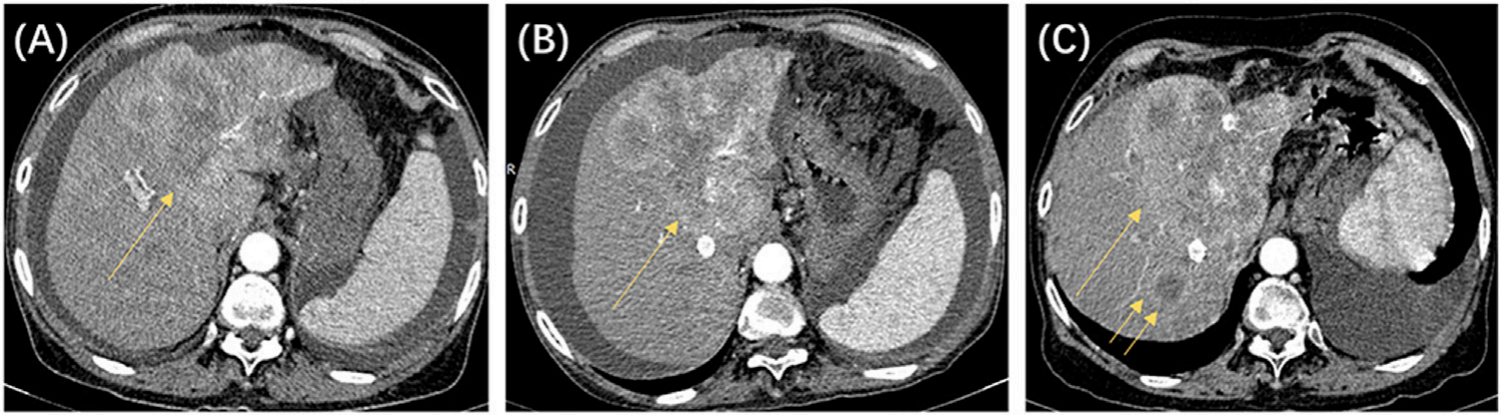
The patient was a 64-year-old woman, with a clinical diagnosis of hepatocellular carcinoma, Barcelona Clinic Liver Cancer stage B, and pre-treatment hepatic function of the Child–Pugh grade (B). The above shows the contrast-enhanced CT images before and after locoregional interventional therapy. (A) Image taken before the initial drug-eluting beads transcatheter arterial chemoembolization (DEB-TACE) procedure, showing that the left liver lobe is full of blood-supplying, space-occupying lesions (single arrow). (B) CT image 8 weeks after the initial TACE procedure. Significant enhancement was still seen for the target tumor (single arrow). The target tumor was assessed to be stable disease based on modified response evaluation criteria in solid tumors (m-RECIST) criteria. (C) CT image 8 weeks after the second TACE procedure. Significant enhancement was still seen for the target tumor (single arrow), and new intrahepatic nodules were seen (double arrows). The target tumor was assessed to be progressive disease based on m-RECIST criteria. According to the untreatable progression (UP) criteria, the patient did not achieve an objective response after two DEB-TACE treatments and was determined to have UP.
